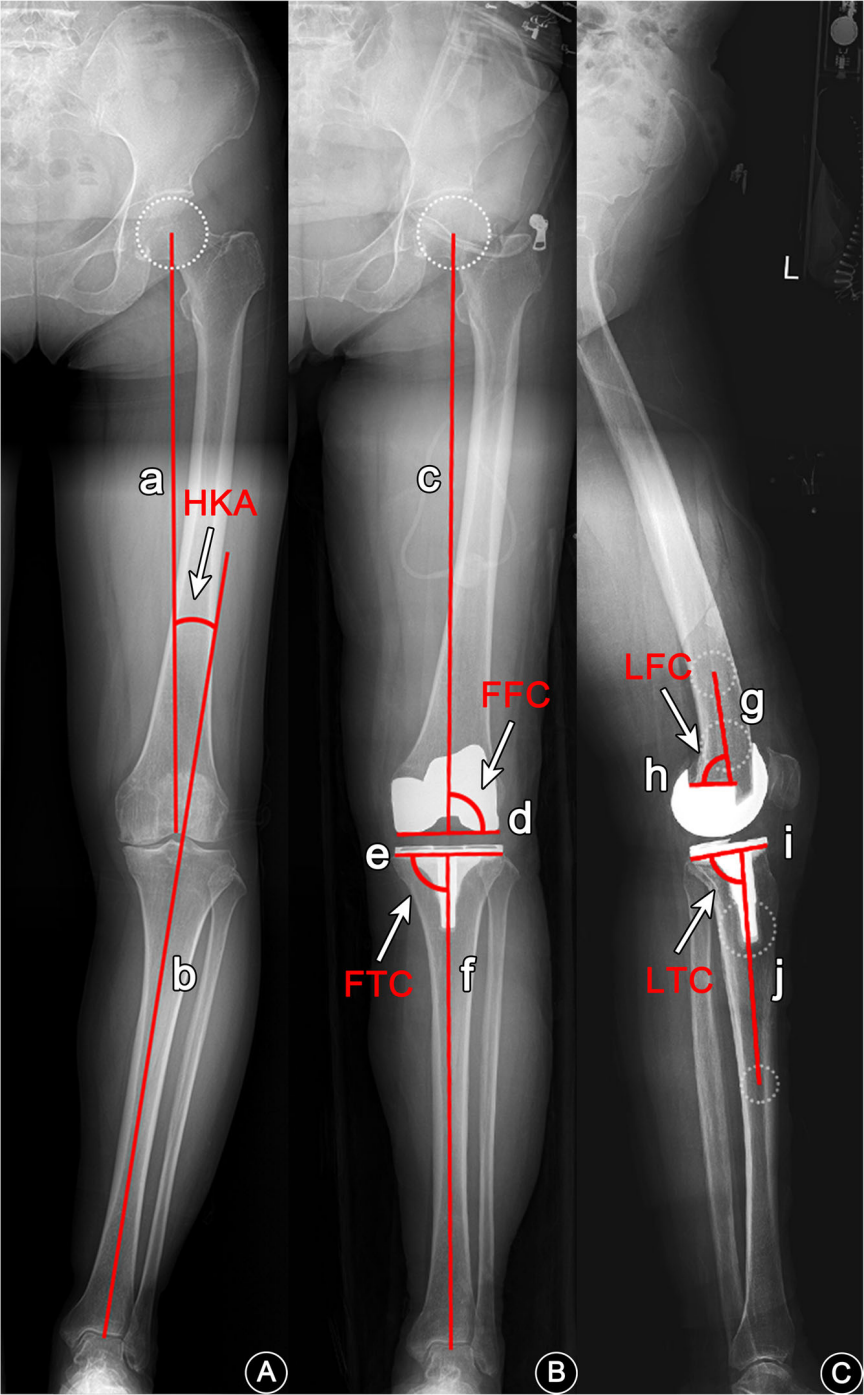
Measurement of HKA, FFC, FTC, LFC, and LTC. HKA hip-knee-ankle angle, FFC frontal femoral component angle, FTC frontal tibial component angle, LFC lateral femoral component angle, LTC lateral tibial component angle. a Line a was the femoral mechanical axis, and line b was the tibial mechanical axis; the acute angle formed between them was recorded as the preoperative HKA. b Line c was the postoperative femoral mechanical axis, line d was the line across the bottom of the femoral condyles, line e was the line across the bottom of the tibial plateau on the anteroposterior radiograph, and line f was the postoperative tibial mechanical axis; the lateral angle between line c and line d was recorded as frontal femoral component angle (FFC), the medial angle between line e and line f was recorded as frontal tibial component angle (FTC), and the acute angle between line c and line f was recorded as the postoperative HKA. c Line g was the line connecting the center points of the femoral shaft at 0 cm and 5 cm above the implant, line h was the line across the bottom of the femoral implant, line i was the line across the bottom of the tibial plateau on the lateral radiograph, and line j was the line connecting the center points of the tibial shaft at 5 cm and 15 cm below the joint line; the posterolateral angle between line g and line h was recorded as lateral femoral component angle (LFC), and the posterolateral angle between line i and line j was recorded as lateral tibial component angle (LTC)
